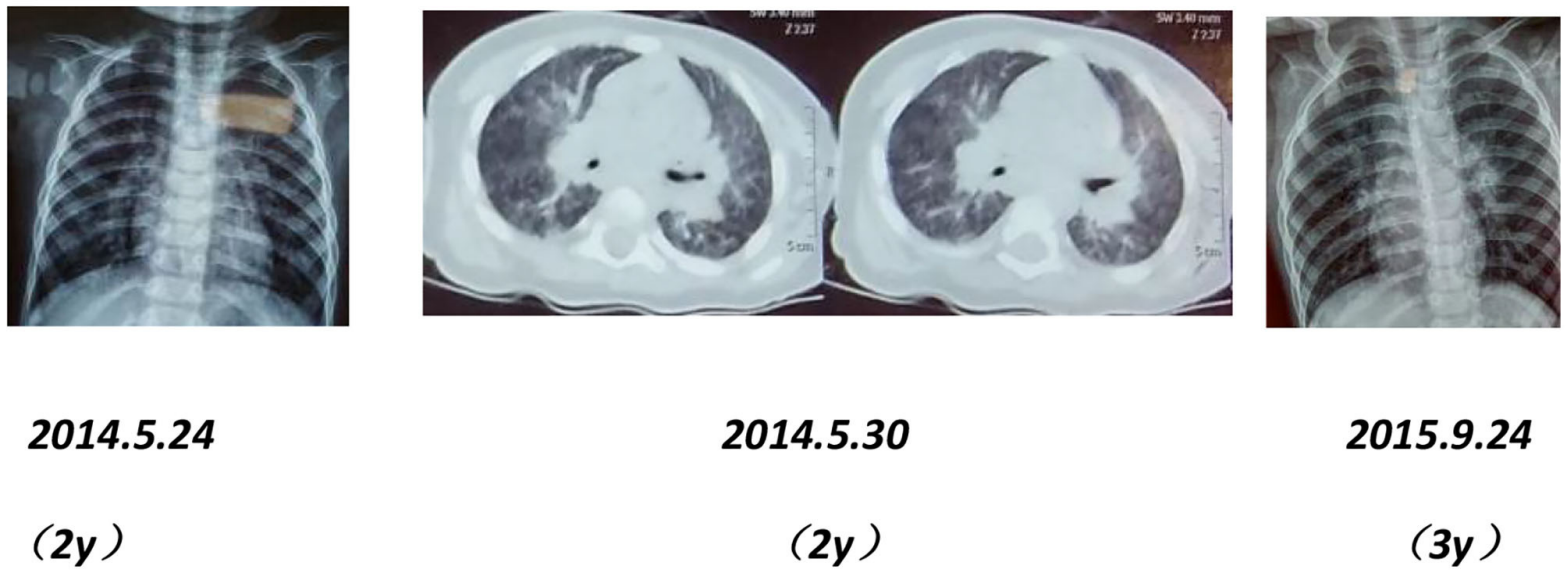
Chest X-rays and CT scans of the proband's younger brother show interstitial pulmonary inflammation.
